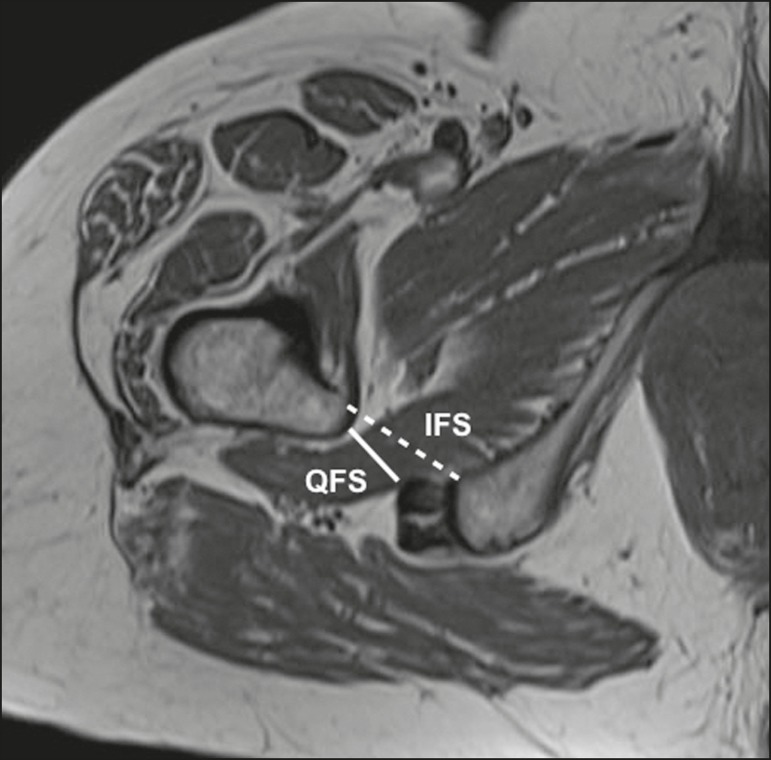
Axial T1-weighted MRI sequence showing the IFS (defined as the shortest
